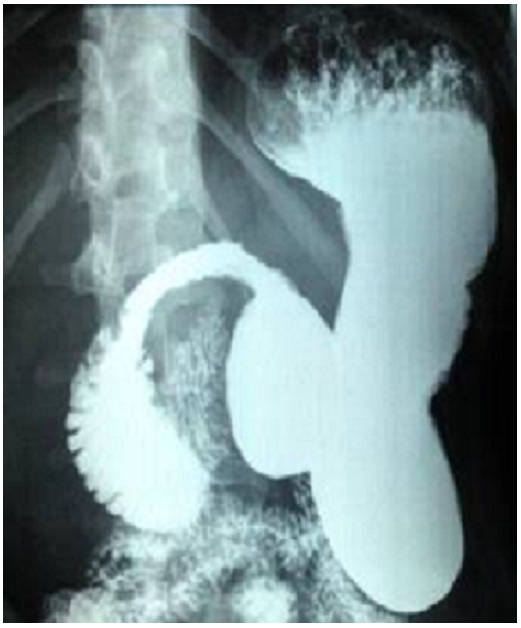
Contrast barium radiography showing an obstructed third part of the duodenum. Proximal to the obstruction a dilated duodenal bulb and stomach was found: classically known as “double bubble” sign
